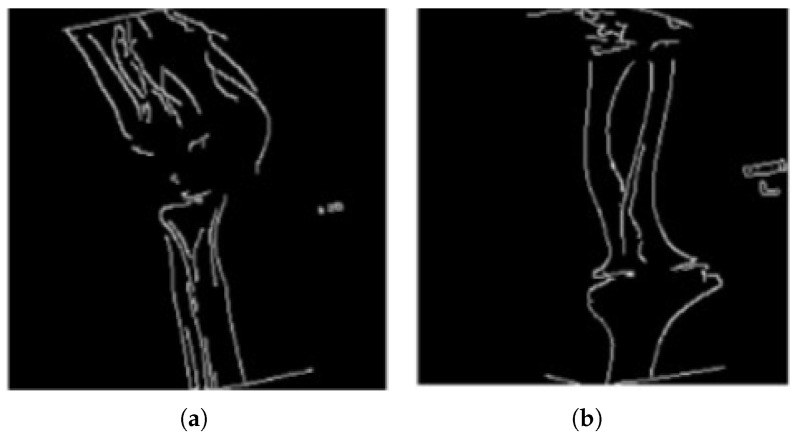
Sample X-ray images processed with Canny edge detection: (a) shows a fractured bone, while (b) shows a normal bone with no fracture.
